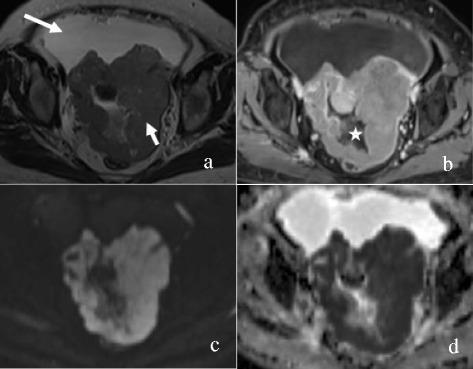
A 67-year-old woman with left-sided HGSC. Axial T2WI (a) demonstrates a solid mass (short arrow) with an irregular shape and a large volume of ascites (long arrow). The mass is markedly enhanced with irregular areas of necrosis (asterisk) on contrast-enhanced T1WI with fat suppression (b), hyperintensity on DWI (c) and hypointensity on ADC map with an ADC value of 0.682 × 10^−3^ mm^2^/s (d).
